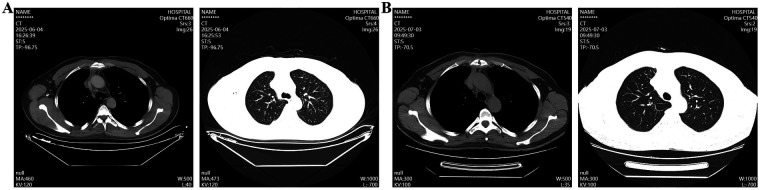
The chest CT scan after the operation indicated that the postoperative condition was good. (A) The results of a plain chest CT scan in June 4, 2025; (B) The results of a plain chest CT scan in July 3, 2025.
